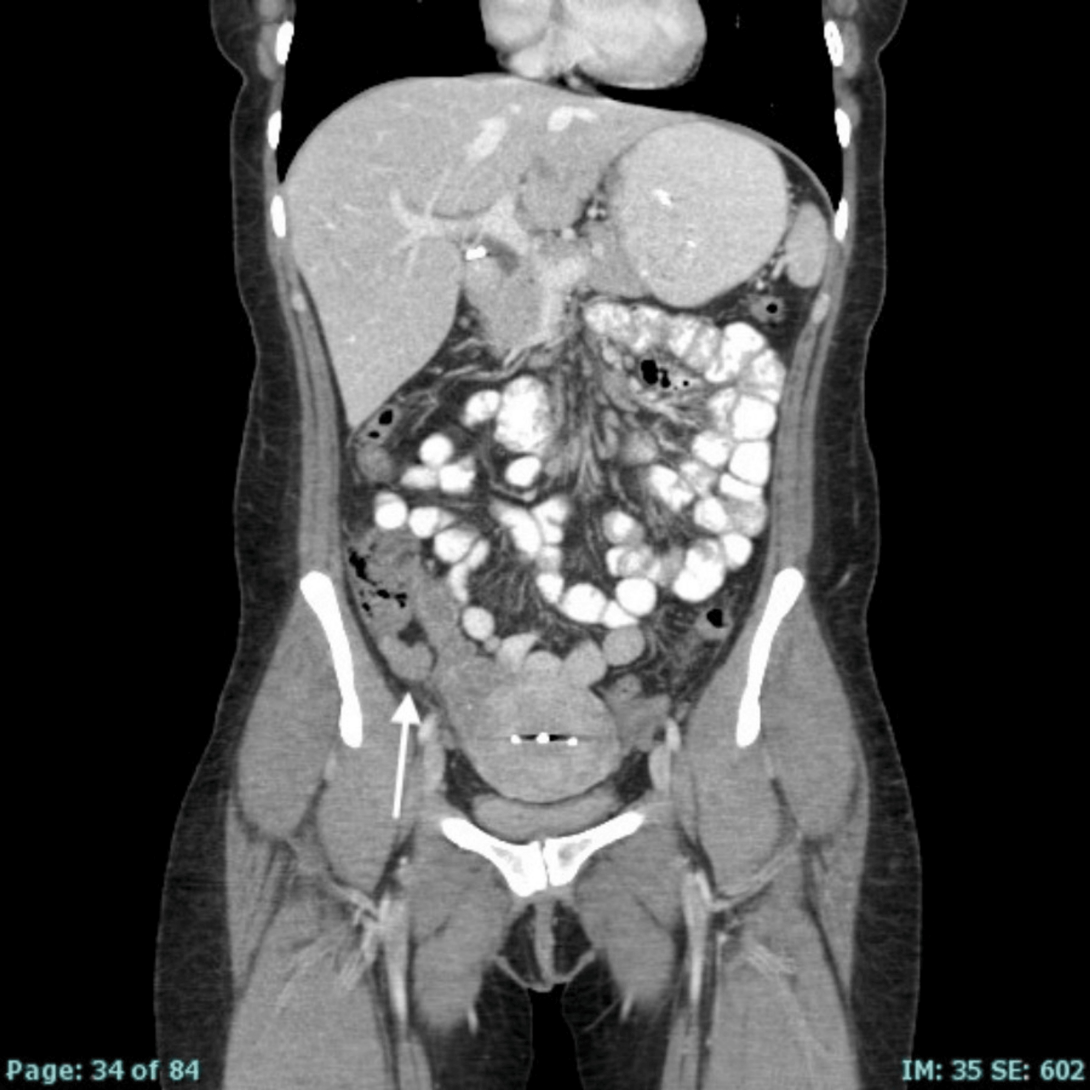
Coronal view of CT of the abdomen and pelvis suggesting thickening of the appendix.
